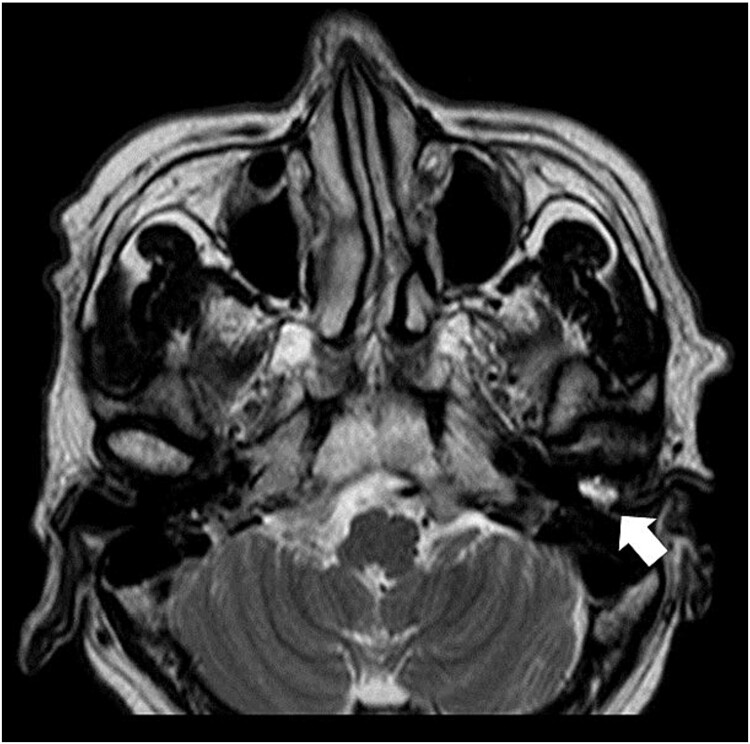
Axial T2-weighted MRI. MRI image shows a high-signal area in the left external auditory canal.
